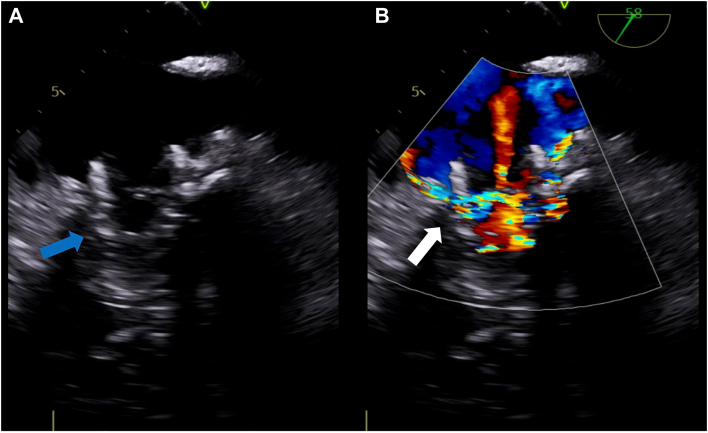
Intraprocedural transesophageal echocardiography after first valve deployment. (A) Transcatheter mitral valve replacement valve migrating toward left atrium (arrow). (B) Severe perivalvular leak (arrow).
